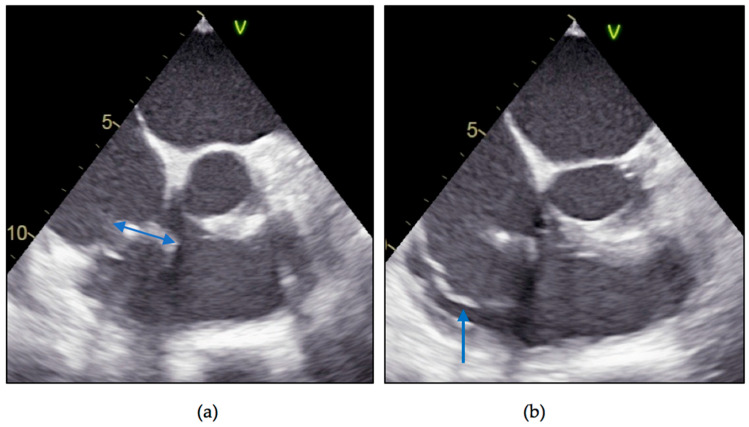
Transesophageal two-dimensional echocardiography: (a) pedunculated mobile mass (blue, double-headed arrow) attached to pacing lead (19/5 mm); (b) posterior leaflet of the tricuspid valve with hypermobility and rupture of chordae (blue arrow).
